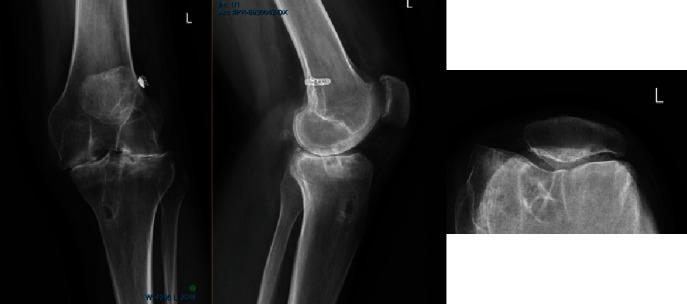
Radiograph of the left knee 7 months later in 2019.
